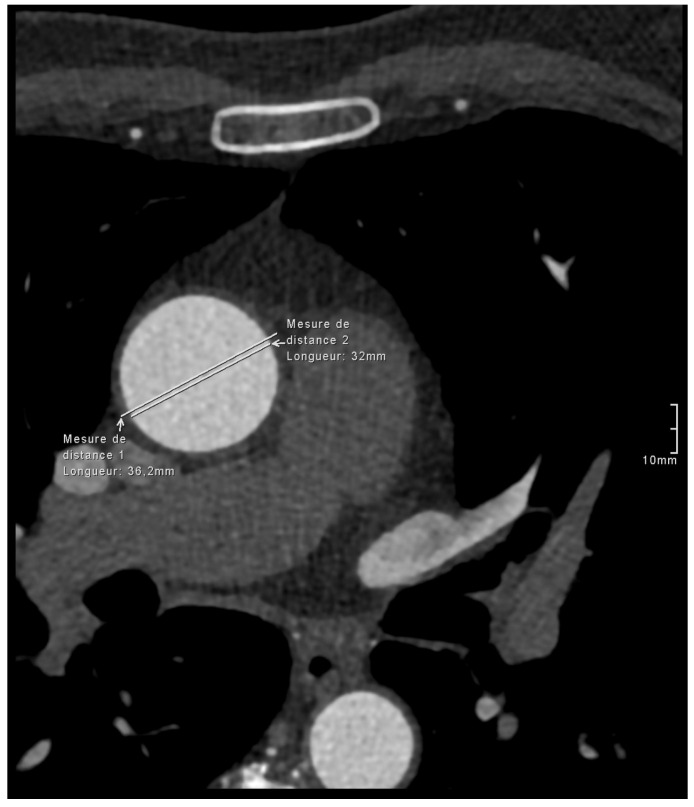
Axial image from a CTA exam of a patient with a thickened aortic wall, thus allowing aortic wall visualization, demonstrating a 4 mm discrepancy between the measurements, including and excluding the aortic wall.
